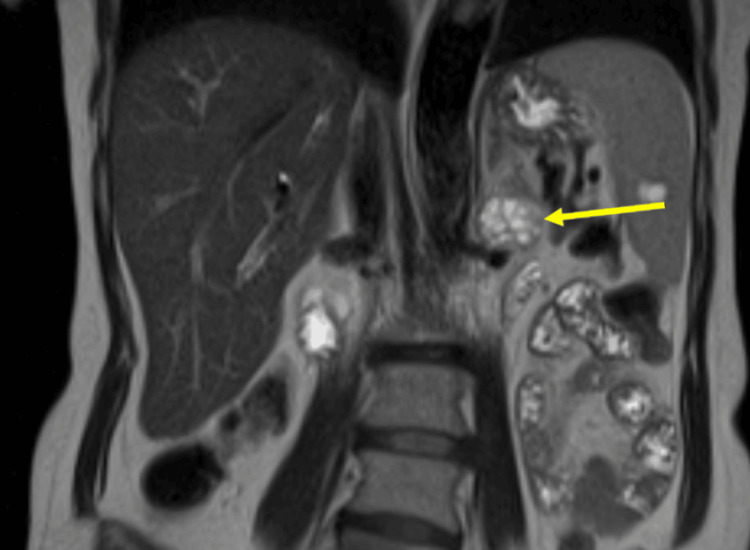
Retroperitoneal mass (yellow arrow) on MRI MRI: magnetic resonance imaging
